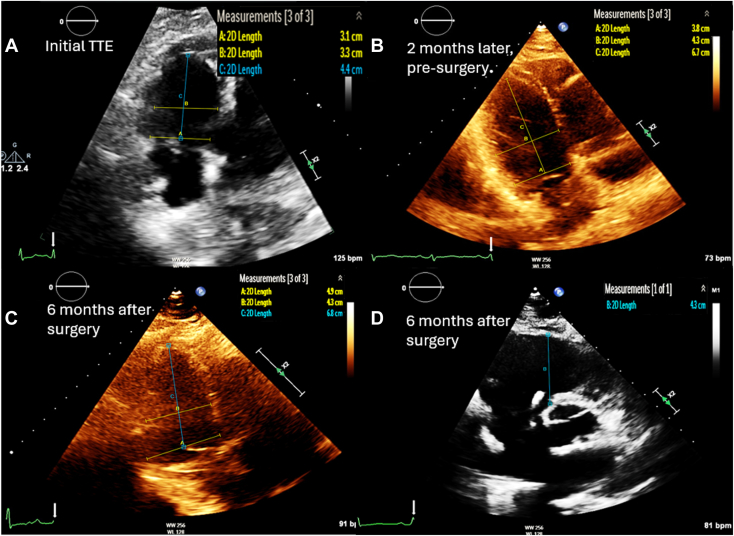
Serial two-dimensional TTE, obtained at baseline, 2 months, and 6 months postoperatively, right ventricular focused apical 4-chamber (A, B and C) and right ventricular short-axis inflow-outflow (D) systolic views, demonstrates progressive dilation of the RV leading to TV replacement surgery, with residual, persistent right ventricular dilation postoperatively.
